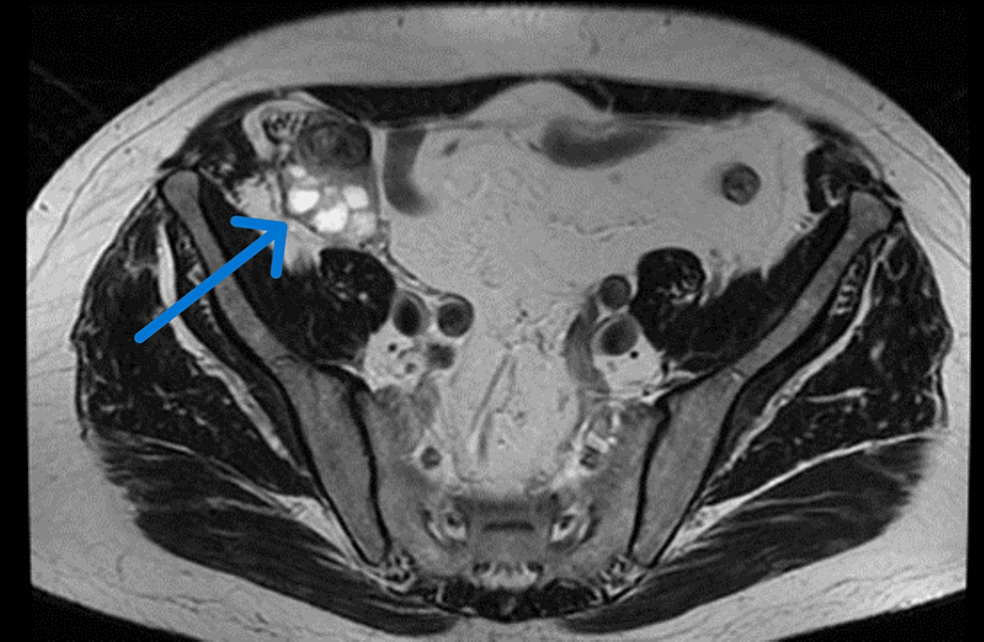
Follow-up MRI (T1-weighted) imaging of the mass. The arrow shows the mass in the right iliac fossa, measuring 5.3 cm in diameter.
